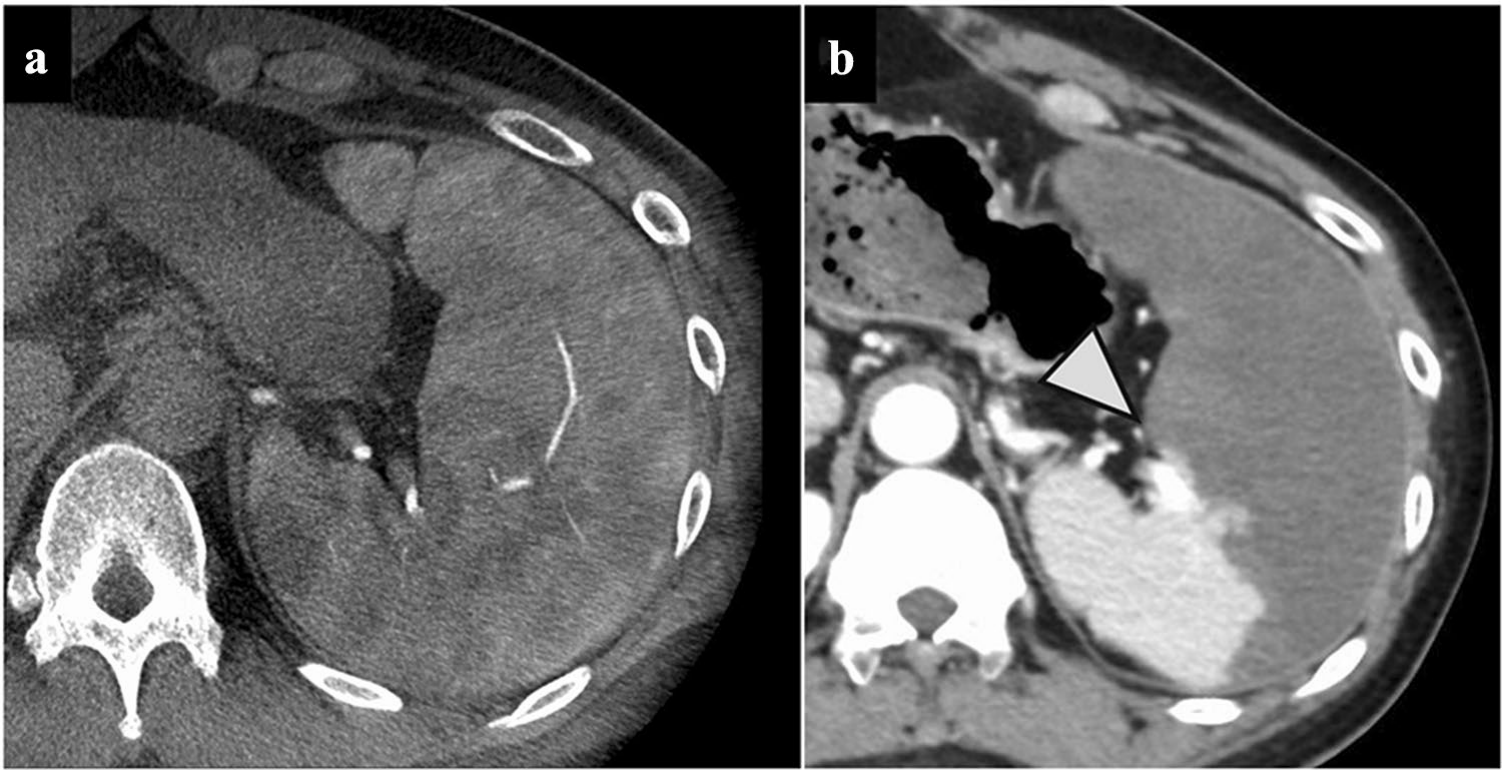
Contrast-enhanced CT during PSE. The middle to lower poles of the spleen were poorly contrasted (a. preoperative CT scan, b. postoperative CT scan; arrowhead: boundary of the infarcted spleen)
